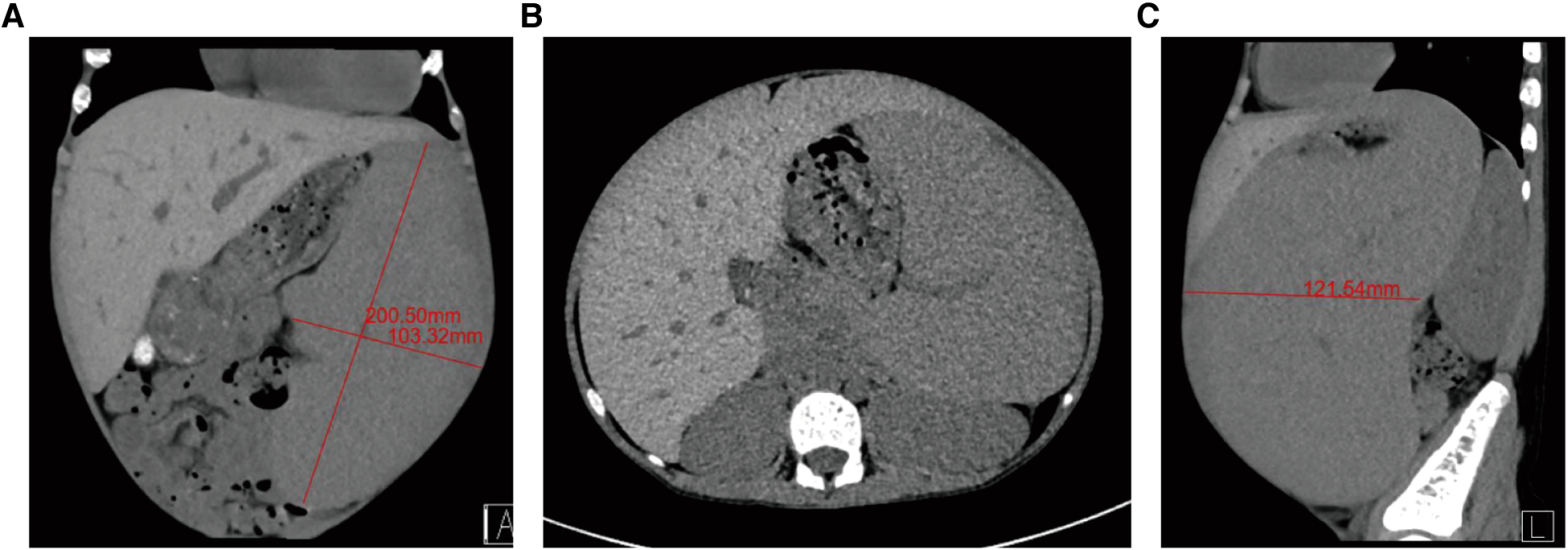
Preoperative computed tomography (CT) images from study participants. (A) Splenic long-axis measurements on the coronal view of abdominal CT, the splenic long-axis larger than 15 cm. (B) Axial CT image showing massive splenomegaly. (C) Sagittal CT image showing massive splenomegaly.
